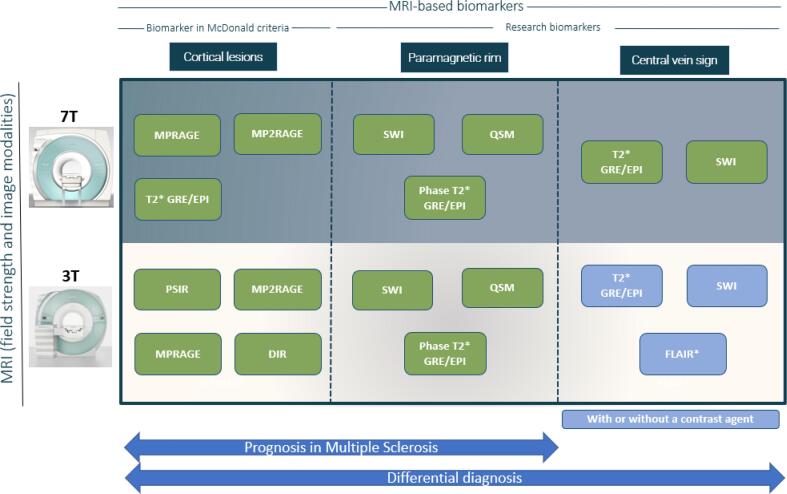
Scheme showing the main MRI sequences used for detecting each biomarker at both 3 T and 7 T.
